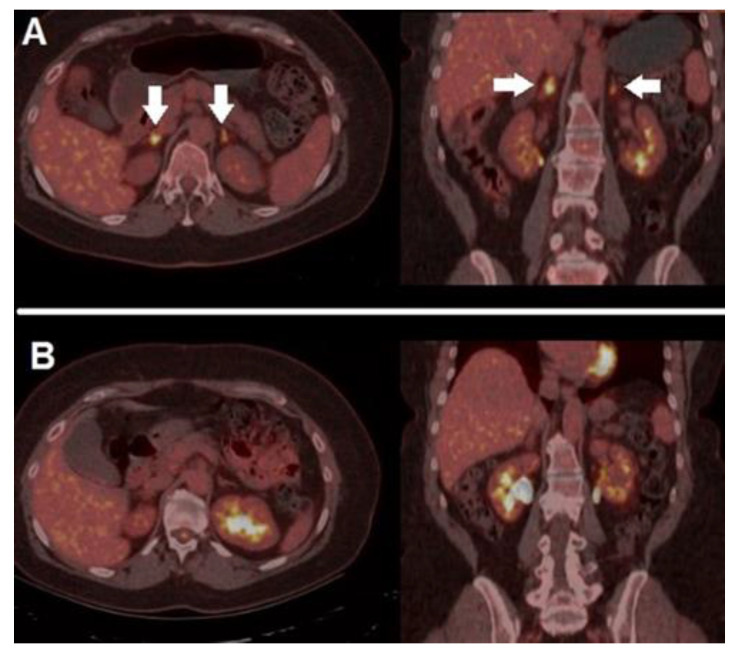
A case of irAEs. A 41-year-old female, previously submitted to excision of a nodular, high-risk MM, started adjuvant immunotherapy with nivolumab. After 4 cycles, she presented mild fatigue and grade 1 hyponatremia and orthostatic hypotension, with reduced level of morning cortisol. ^18^F-FDG PET/CT performed after symptoms’ onset (A) demonstrated bilateral increased tracer accumulation within adrenal glands (white arrows), compatible with the suspicion of immune-related adrenalitis, she discontinued immunotherapy and started on steroid therapy with excellent clinical response. PET/CT, acquired after steroid therapy and symptoms’ regression (B), revealed complete regression of the adrenal glands’ hypermetabolism. After multidisciplinary consensus meeting, immunotherapy was restarted, and no further complications were registered.
